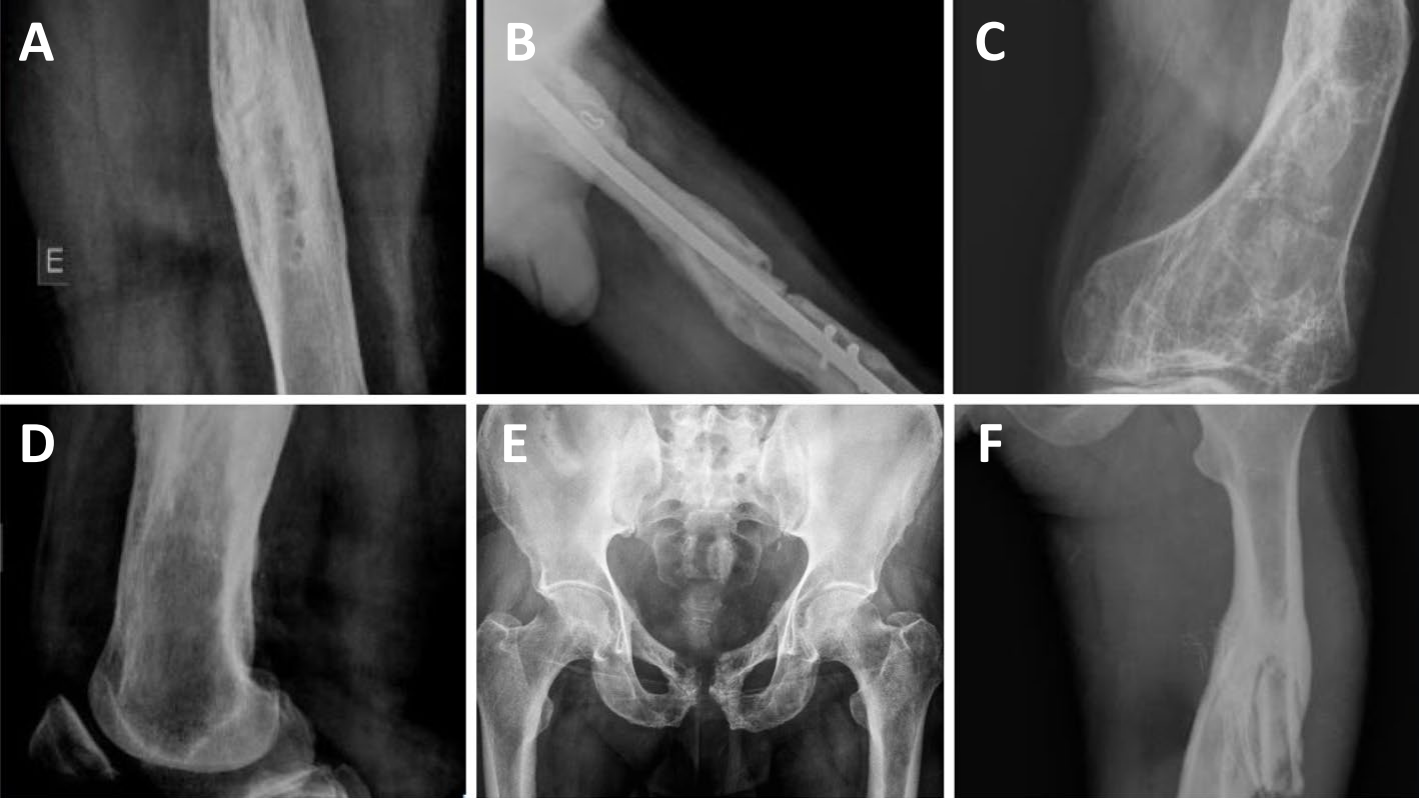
Radiographic findings evaluated in patients with chronic osteomyelitis. A bone sclerosis, B) soft-tissue swelling, C) cortical thinning (with erosion), D) cortical thickening, E) bone destruction, F) sequestrum
